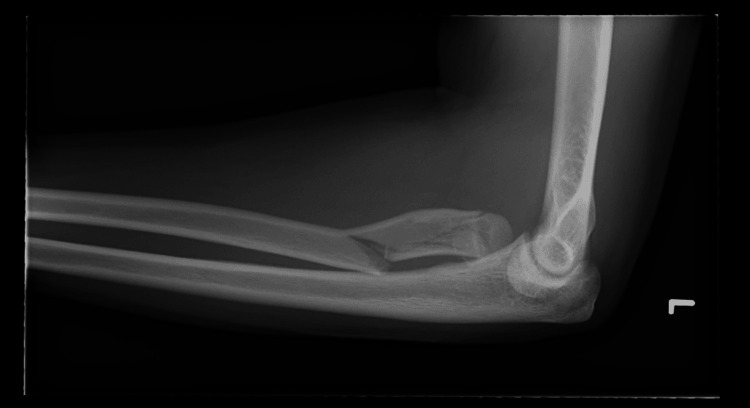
Lateral view of the elbow injury radiograph
